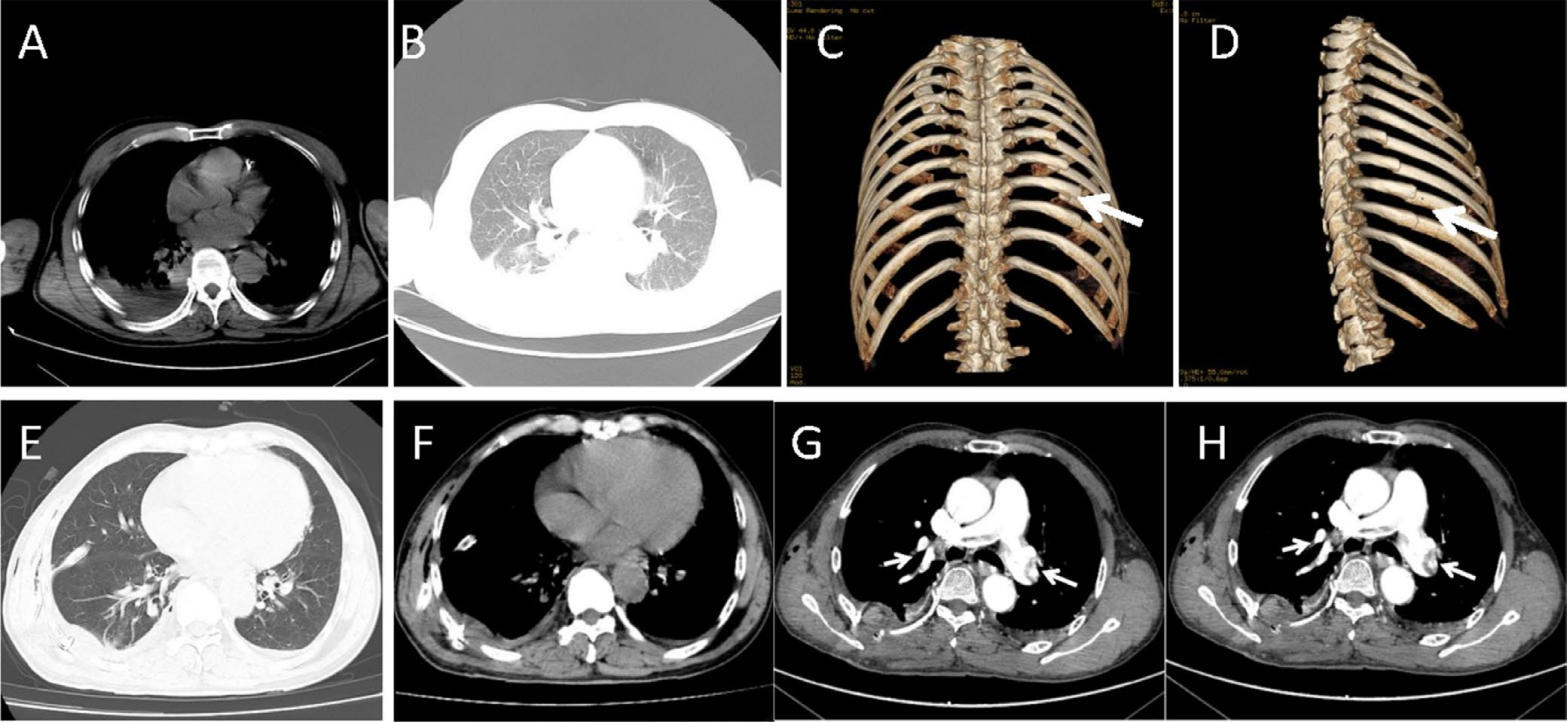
(A) A thoracic CT scan exhibited bilateral pleural effusion with apparent compression and incomplete expansion of the lower lobe of the right lung. (B) The patchy and strip‐like increased density on the back of both lungs was found in the chest CT. (C, D) 3D reconstruction of the chest CT revealed multiple right fractures of the 2nd–10th ribs on the right side with partial dislocation. (E, F) CT of the chest indicated a significant decrease in pleural effusion and a small amount of inflammation in both lungs. (G, H) Pulmonary artery angiography of the CT revealed multiple pulmonary embolisms at the distal end of the left and right main pulmonary arteries and branches of each lobe. Chest CT revealed exudative lesions of both lungs with atelectasis in the lower lobe of the left lung and a small amount of pleural effusion in the lungs.
